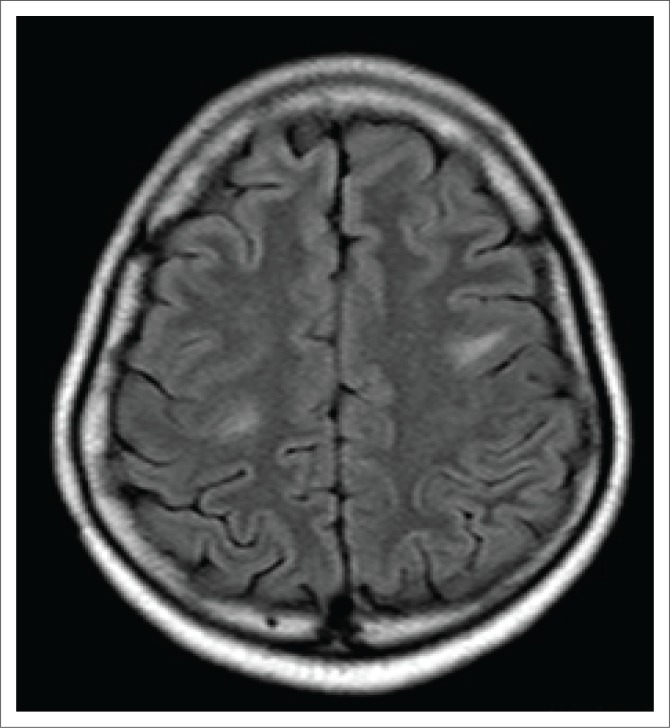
Axial T2-weighted image with hyperintense subcortical white matter lesions.
